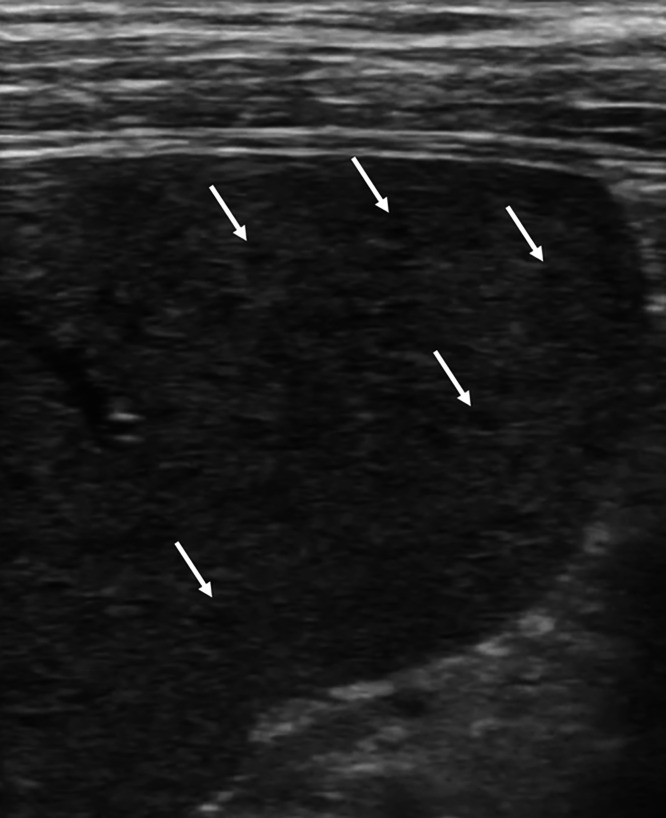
Ultrasound of the spleen revealed numerous 1- to 2-mm hypoechoic lesions (arrows) in a “sponge-like” pattern.
